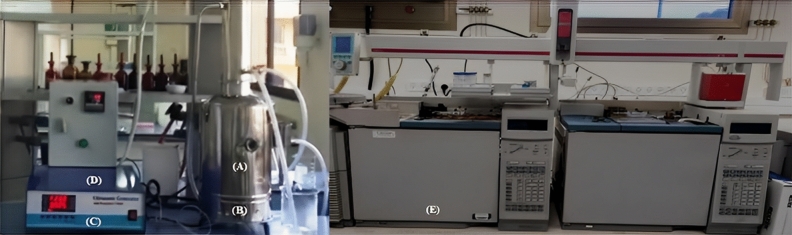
Dual-function GC/MS optimized ultrasound-assisted hydrodistillation prototype (DF-GC/MS-HUS). (A) Modified Clevenger hydro-distillator (upper-part). (B) Imbedded ultrasound transducer (lower-part). (C) Ultrasound control. (D) Central Control. (E) GC–FID–MS.
